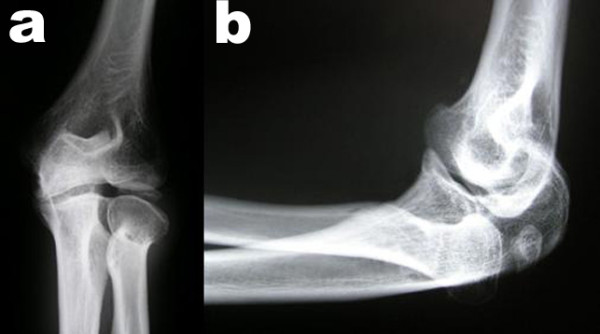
X-rays of the left elbow (a: anterior posterior and b: lateral view) demonstrating dysplastic deformity of the radial head.
